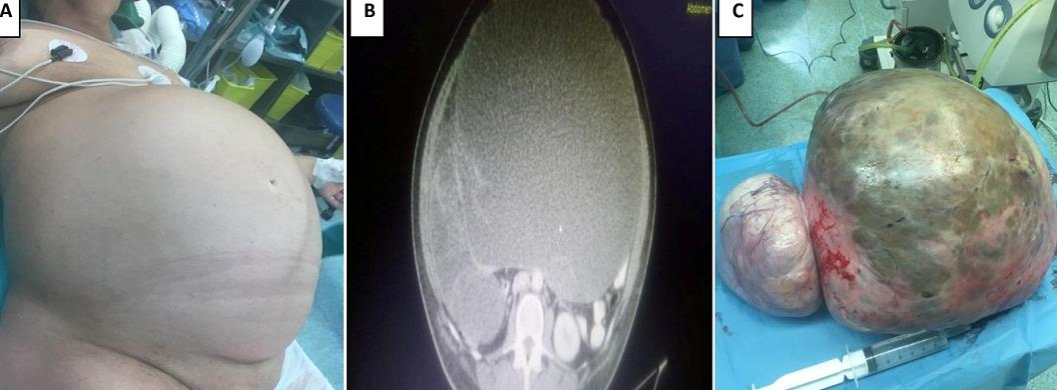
A) giant abdominal mass; B) abdominal and pelvis CT revealed a giant mass arising from the right adnexa; C) completely resection of the ovarian mass that weighed 15,4kg and measured 44x39x19cm
